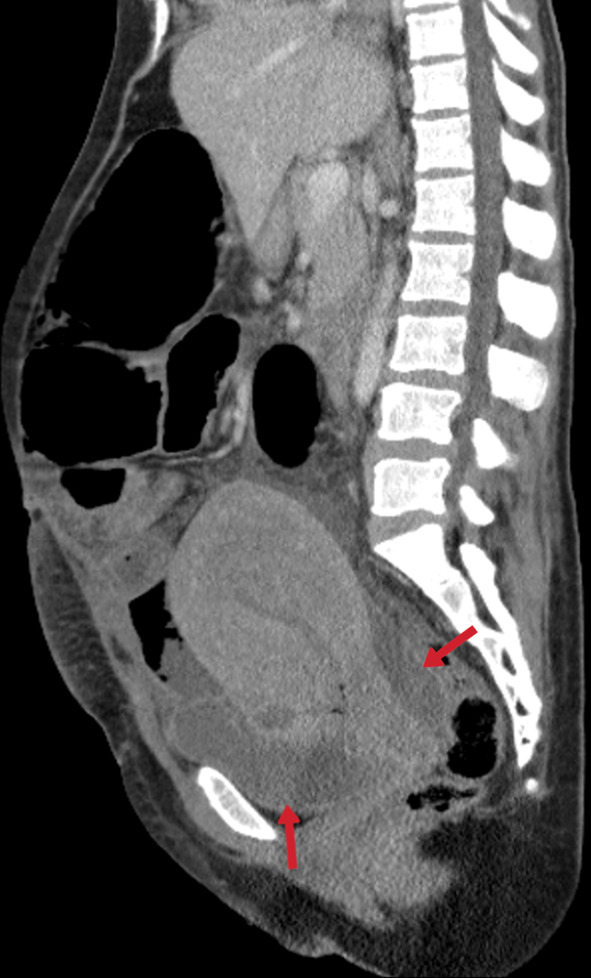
Sagittal-reformatted abdomen CT image of a 22-year-old female with lower abdominal pain and fever obtained after C/S surgery. The uterus is enlarged due to prior pregnancy. Free fluid is noted in the pelvis (arrows). Tuberculous pelvic inflammatory disease
